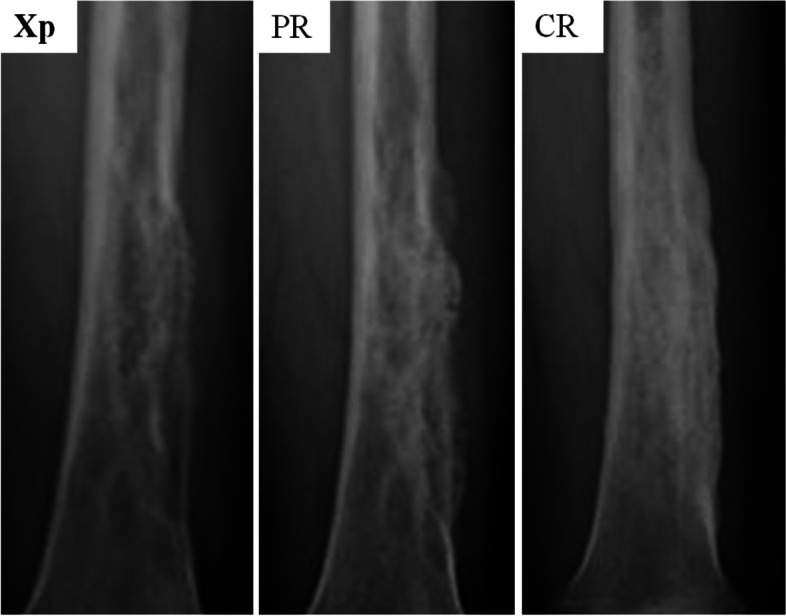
Representative radiographs showing evaluation criteria. Radiographs obtained before treatment show lytic metastasis. After some treatment, the radiographs showed “partial filling in,” categorized as partial response (PR), and “complete filling in,” categorized as complete response (CR)
